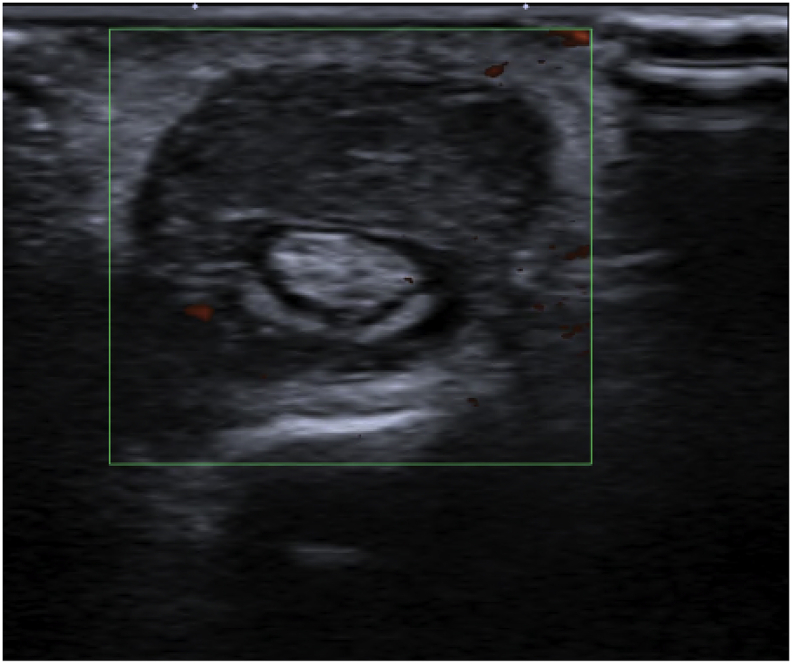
Transverse ultrasound view showing hypoechoic lesion volar to flexor digitorum tendon and tenosynovitis of 5th finger.
